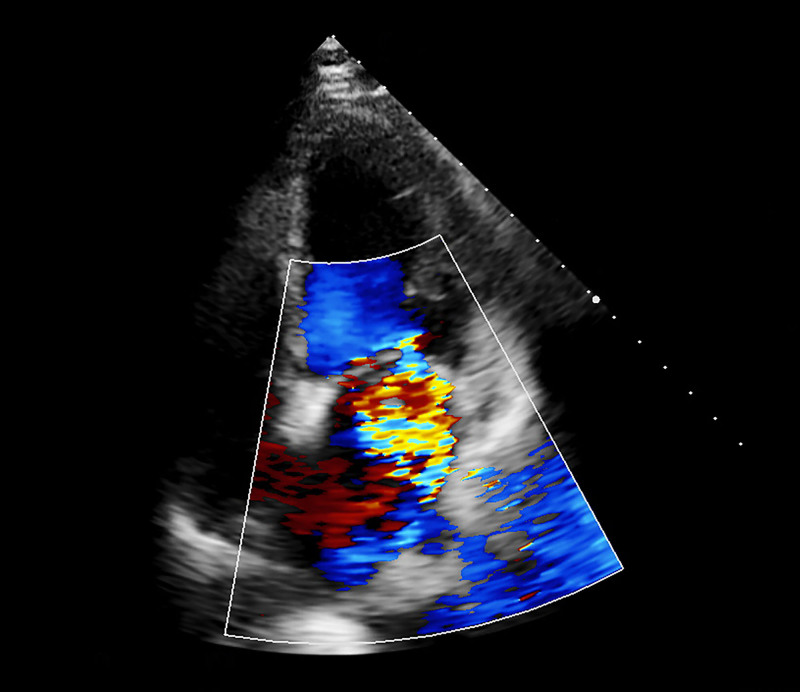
Transesophageal echocardiography still image demonstrating severe mitral regurgitation.
